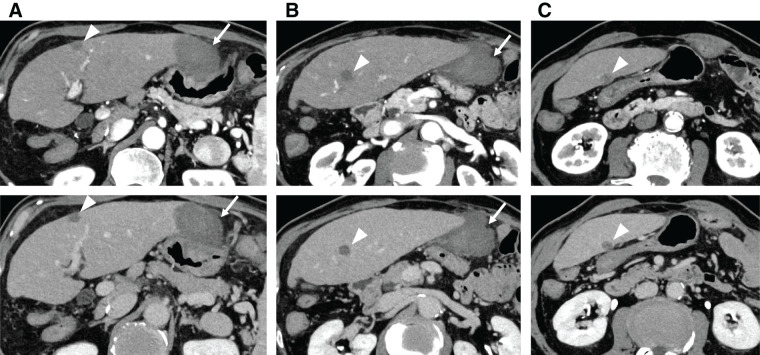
Abdominal contrast-enhanced CT images taken after the first cycle of atezolizumab plus bevacizumab therapy (above: arterial phase images; below: equilibrium phase images). No changes are observed in tumor size (arrowheads); however, the vascularity of all tumors is markedly suppressed (A–C). Ruptured HCC is identified in the lateral portion of S2 (arrows); however, the hematoma is localized in the subcapsular region, and no extravasation or pseudoaneurysm is observed (A, B). HCC, hepatocellular carcinoma
